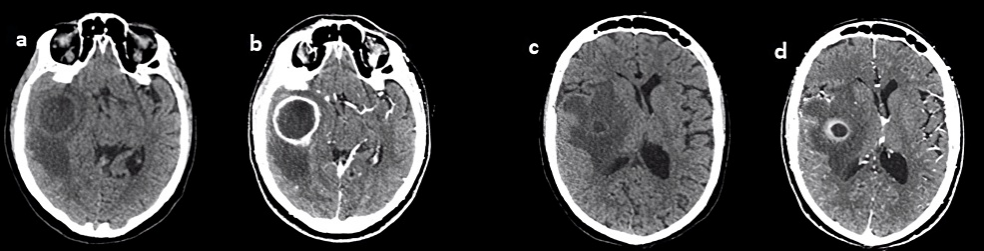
Initial brain computed tomography, pre- (a, c) and post (b, d) contrast, demonstrating two ring-enhancing lesions in the right temporal and parietal lobes.
